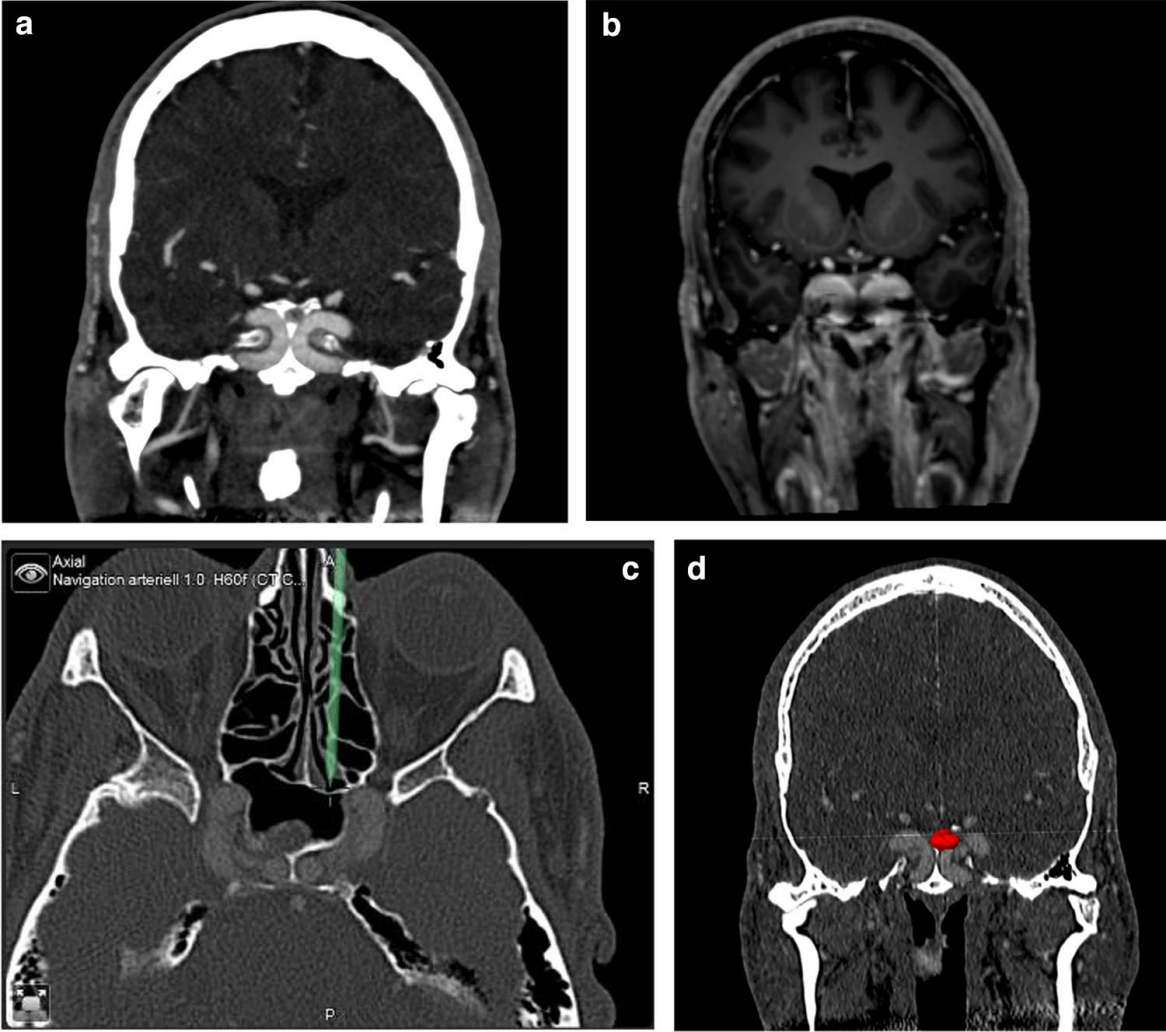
MRI and CT angiography demonstrating a a 57-year-old male acromegalic patient with a narrow intercarotid distance at paraclival and intercavernous space (kissing carotid arteries), b MRI angiography demonstrating the poor spatial resolution in this region, c the protrusion of the carotid artery into the sphenoid sinus with an extension up to the rostrum of the cavernous sinus and d CT angiography with marked STH-producing microadenoma
